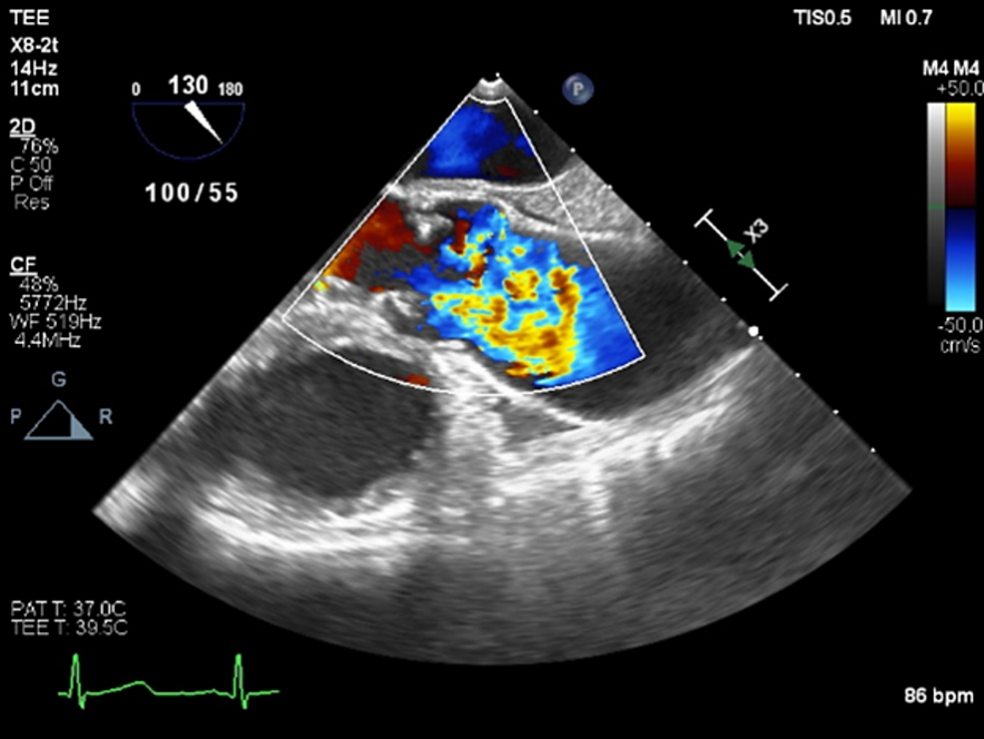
Transesophageal echocardiogram (TEE) demonstrating pulmonic valve insufficiency
